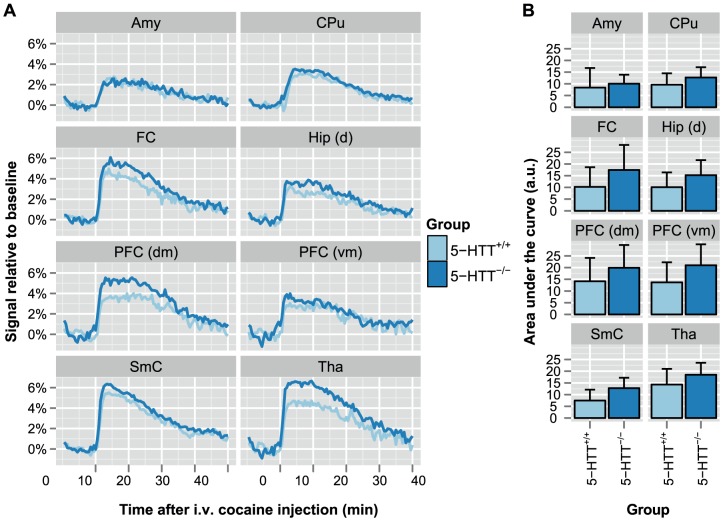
Cocaine-induced activation responses from seed-based analysis of pharmacological MRI. BOLD-based pharmacological MRI was performed for 50 minutes, with 1 mg/kg cocaine injected intravenously after 10 minutes. (A) The raw signal time-courses after preprocessing, and (B) the area-under-the curve of gamma-variate functions obtained with a Bayesian fitting routine, are displayed for a selection of seed regions. *Amy*: basal amygdaloid nuclei; *CPu*: caudate-putamen; *FC*: frontal cortex; *Hip (d)*: dorsal hippocampus; *PFC (dm)*: dorsomedial prefrontal cortex; *PFC (vm)*: ventromedial prefrontal cortex; *SmC*: somatosensory cortex; *Tha*: thalamic nuclei.
